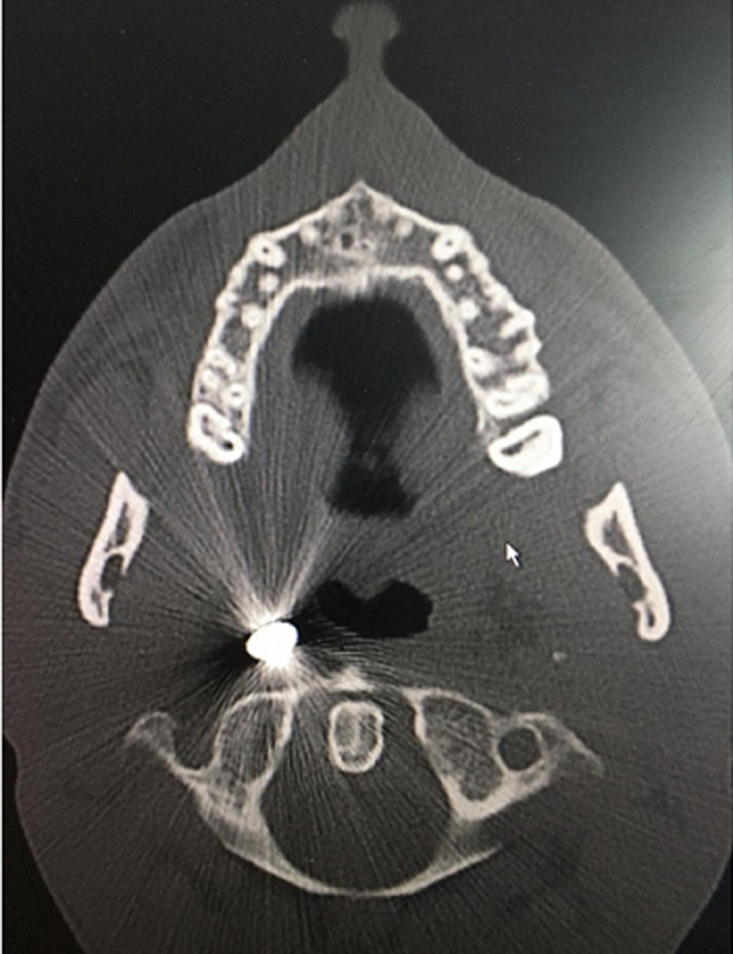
axial view of the computed tomography scan
